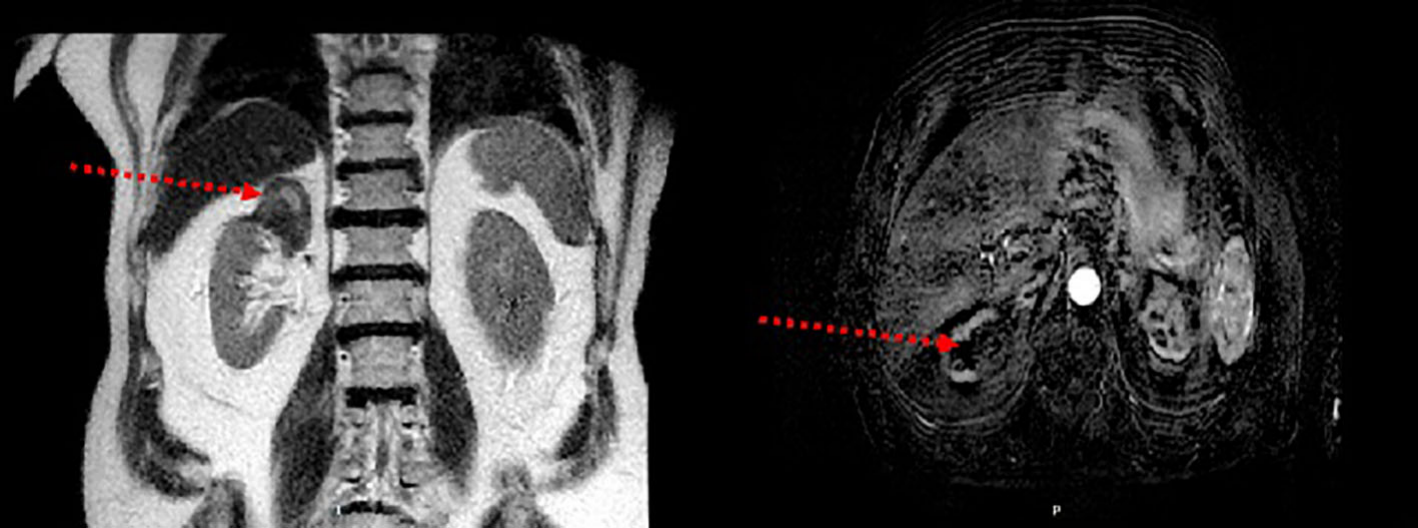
Post-procedure magnetic resonance imaging. (Left picture) The right upper polar cryoablation zone (see arrow) is in discreetly heterogeneous T2 hyposignal. (Right picture) T1 sequence injected at subtracted arterial time showing no nodular enhancement over the cryoablation area (see arrow).
